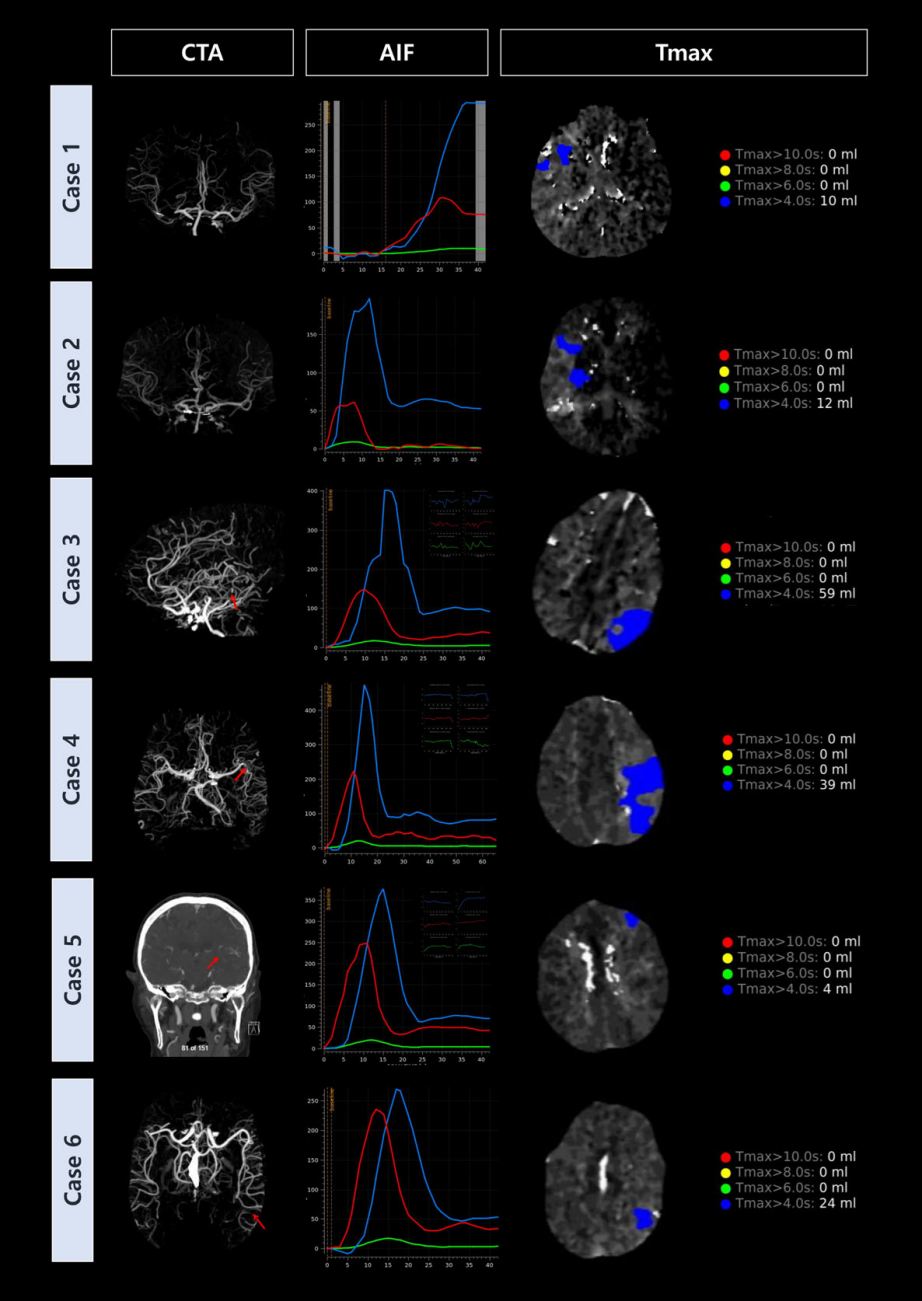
Six patients who did not demonstrate concordance between CTP and CTA. In all patients, if abnormality for Tmax threshold is set above 4 sec, perfusion deficit could be detected. Case 1: Right MCA‐M1 occlusion. The early truncation of AIF and VOF curves limited the accurate estimation of Tmax, contributing to the mismatch threshold fallacy. Case 2: Right MCA‐M1 occlusion. Insufficient time for baseline CT attenuation affected the precise estimation of Tmax, highlighting the importance of a 5–10 sec pre‐contrast baseline (indicated by two parallel dash lines before the slope) for optimal scans. Case 3: Left MCA‐M2 occlusion. Inadequate baseline CT attenuation and motion artifact during scanning compromised the accurate estimation of Tmax, contributing to the threshold fallacy observed. Case 4: Left MCA‐M2 occlusion. Similar challenges with baseline CT attenuation and motion artifact affected the reliability of Tmax estimation, influencing the discordance between CTP and CTA findings. Case 5: Left distal MCA‐M3 occlusion. Challenges with baseline CT attenuation and motion artifact during the scan compromised the accurate estimation of Tmax, contributing to the observed threshold fallacy. Case 6: Left distal MCA‐M3 occlusion. Insufficient baseline CT attenuation affected the precise estimation of Tmax, highlighting another instance of the threshold fallacy in CTP imaging. CTP, CT perfusion; CTA, CT angiography; Tmax, maximum contrast transit time; MCA, middle cerebral artery; AIF, arterial input function; VOF, venous output function.
